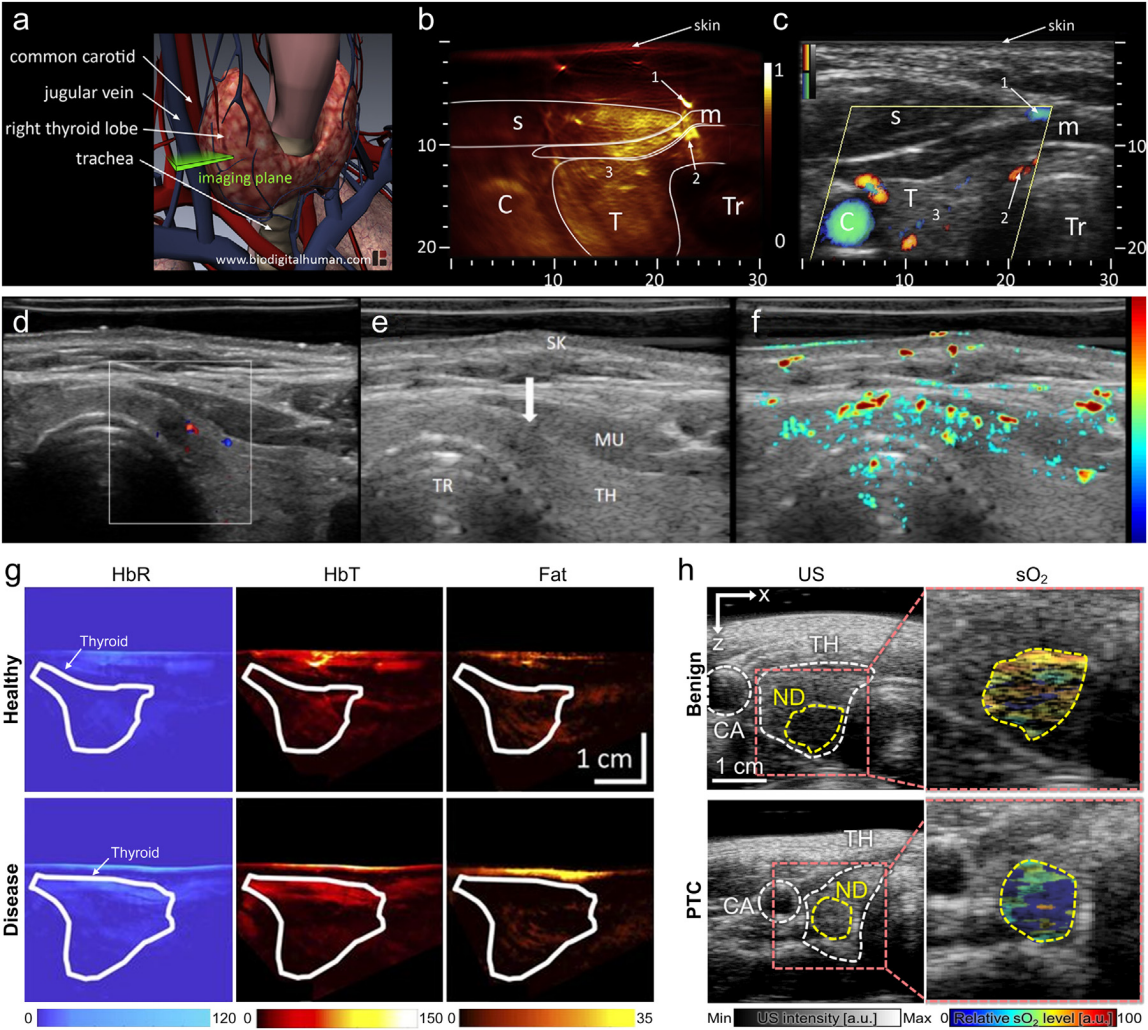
Photoacoustic and ultrasound imaging of the thyroid. (a) Anatomy of thyroid gland including cardio-vascular and respiratory system; the cross-sectional 2D imaging plane is highlighted in green. (b-c) Photoacoustic and ultrasound cross sections of the left thyroid lobe of the first volunteer [71]. C: Carotid, T: Thyroid, Tr: Trachea, s: sternocleidomastoid muscle, m: infrahyoid muscle; axes in mm. Color Doppler flow imaging ultrasound (d), gray scale US B-scan (e) and PA/US (f) imaging results for a left lobe papillary thyroid cancer (PTC, Follicular variation) with the largest diameter of 3 mm [138]. (g) Example pseudo color-coded MSOT images of HbR, HbT, and fat of Graves’ disease and healthy thyroid tissue [139]. Images show higher HbR and HbT and lower fat content in Graves’ disease than in healthy tissue. ROIs transferred from corresponding ultrasound images are shown to localize investigated thyroid lobe. (h) The *in vivo* PAUS images and multispectral PA signals acquired from one benign patient and one PTC patient patients [140].
